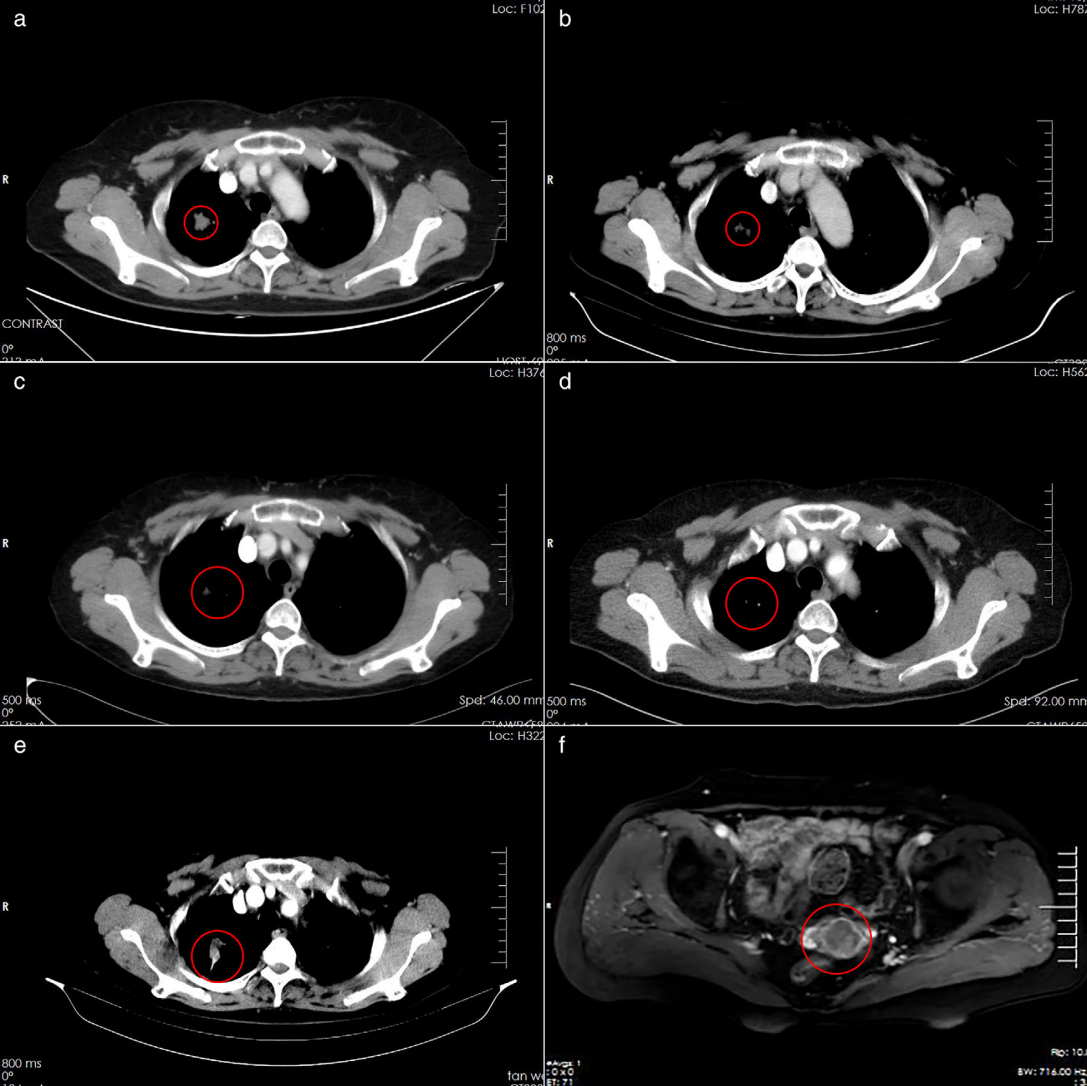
(a) and (b) Imaging before and after gefitinib treatment (a) on March 5, 2016, and (b) April 23, 2016. (c) and (d) Imaging before and after treatment with osimertinib (c) on March 22, 2018 and (d) on June 25, 2018. (e) Chest computed tomography (CT) scan on March 22, 2020. (f) Pelvic scan on April 26, 2020
